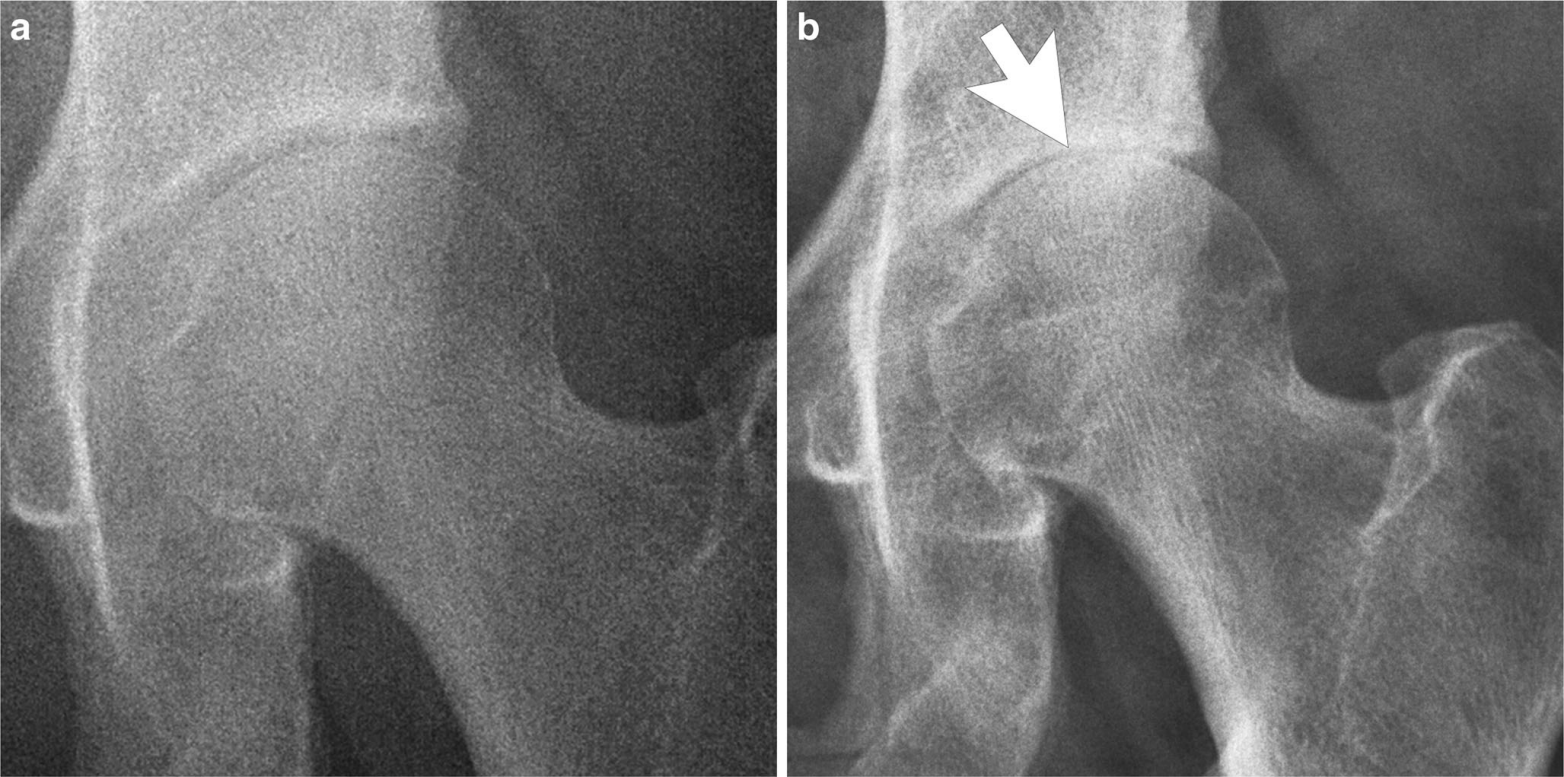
Postsurgical chondrolysis. A 51-year-old woman with a labral tear. Preoperative radiographs were performed elsewhere, and were reportedly normal. She had severe, progressive pain following surgery that was not relieved by narcotic medication. Joint aspiration was negative for infection. a AP weight-bearing radiograph 4 months postoperatively shows mild joint space narrowing. b AP weight-bearing radiograph 8 months after arthroscopy shows progressive, uniform joint space narrowing (*arrow*), but no osteophytes. This radiographic finding is indicative of chondrolysis or infection or inflammatory arthritis
